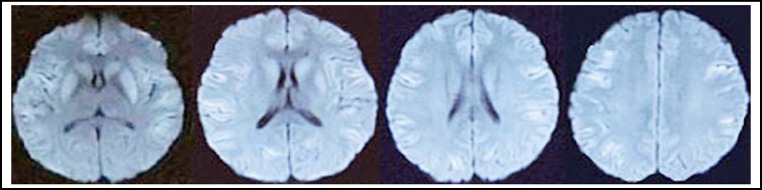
DWI (2013-05-31) showed multiple abnormal signals in bilateral hemisphere and symmetric abnormal signal in bilateral caudate nucleus and putamen
